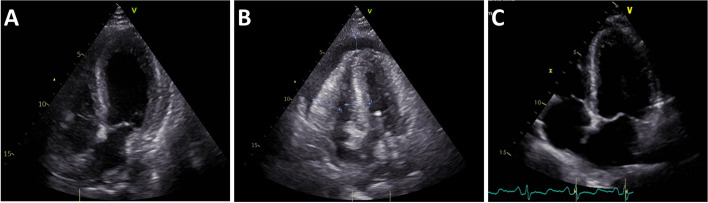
Transthoracic echocardiography. A Day 1. Severely impaired cardiac function, left ventricular ejection fraction ~ 20%, interventricular septum thickness (IVS) 14 mm, circular pericardial effusion (< 8 mm end-diastolic). B Day 2. IVS 21 mm, lateral wall thickness 25 mm, pericardial effusion (11 mm). C Day 15. Normalized cardiac function, left ventricular ejection fraction 65%, IVS 8 mm, no pericardial effusion.
